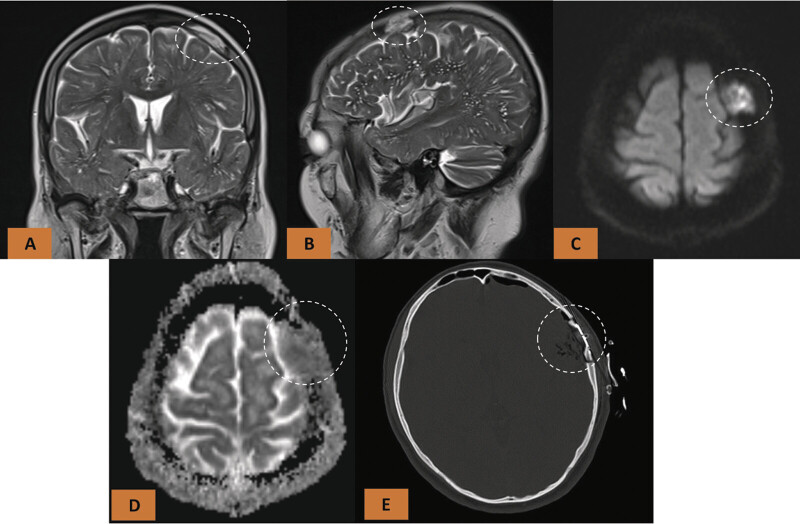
Left frontal dermoid cyst. A non-expansile iso/hyperintense cystic lesion is noted on coronal and sagittal T2-weighted MR images (
